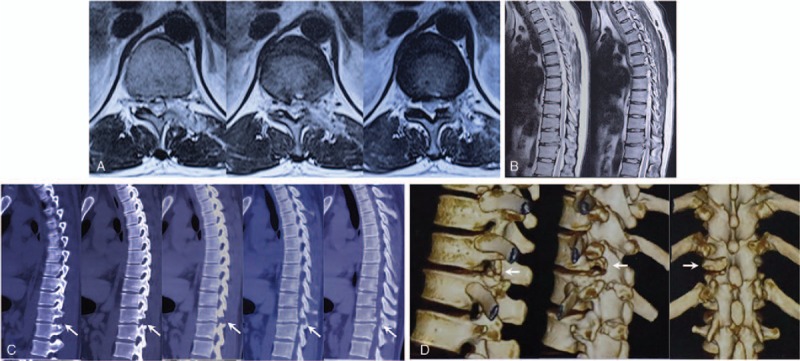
Post-operation imaging revealed satisfying decompression on T11–12. Magnetic resonance imaging demonstrated restored spinal canal and postoperative change of the disc and laminar (A and B). Computed tomography scan displayed that part of the facet and laminar was resected, which provided adequate vision and space for decompression (C and D, arrow).
